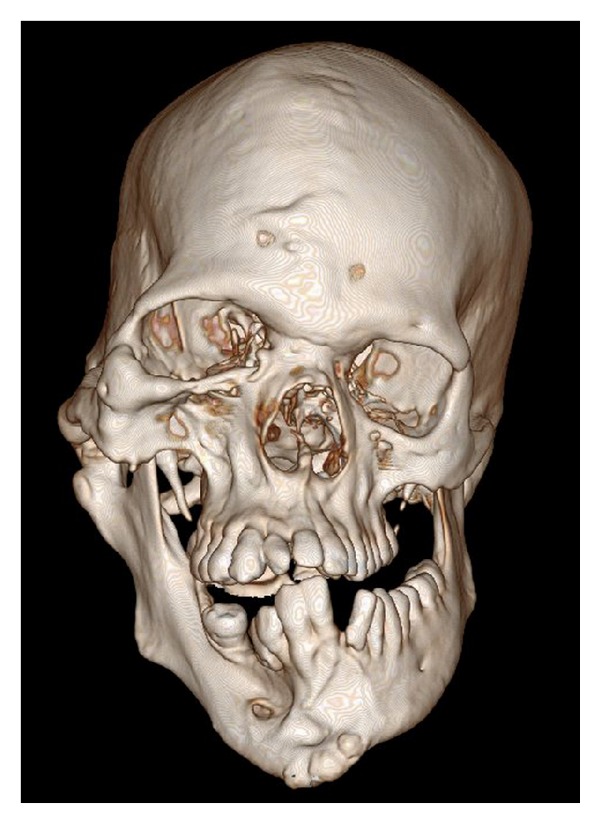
3D volume rendered CT scan showing enlarged mandible, maxilla, and zygoma, with enlarged right mental foramen and teeth of the right side.
